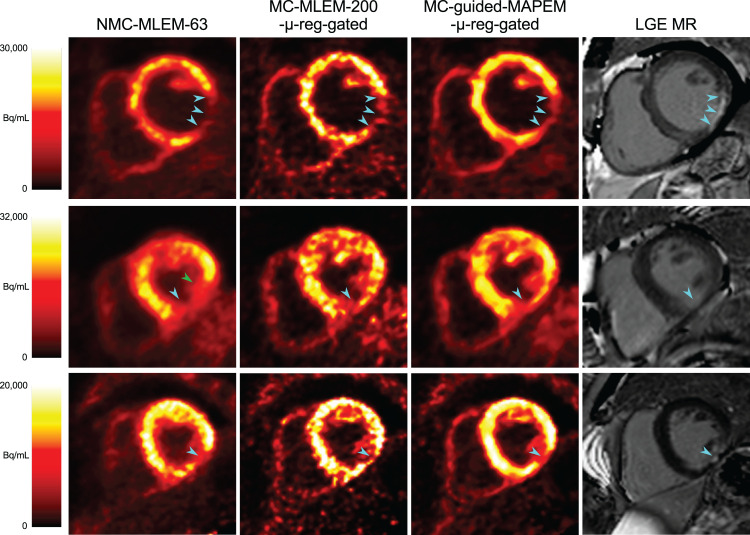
Example short-axis view of reconstructed ^18^F-FDG PET images for 3 selected CTO patients, and corresponding late-gadolinium enhancement (LGE) MR images, showing extent of myocardial scarring. Proposed method improves image quality while maintaining appearance of ^18^F-FDG hypointense defects (cyan arrowheads). MC-MLEM-200-μ-reg-gated images are shown to distinguish effects of motion compensation and guidance. In some cases, uncorrected PET images falsely depict defect as more extensive than it actually is (green arrowhead). LGE images are shown only for comparison and did not provide any information for guided PET reconstructions, which instead used high-resolution CMRA images.
